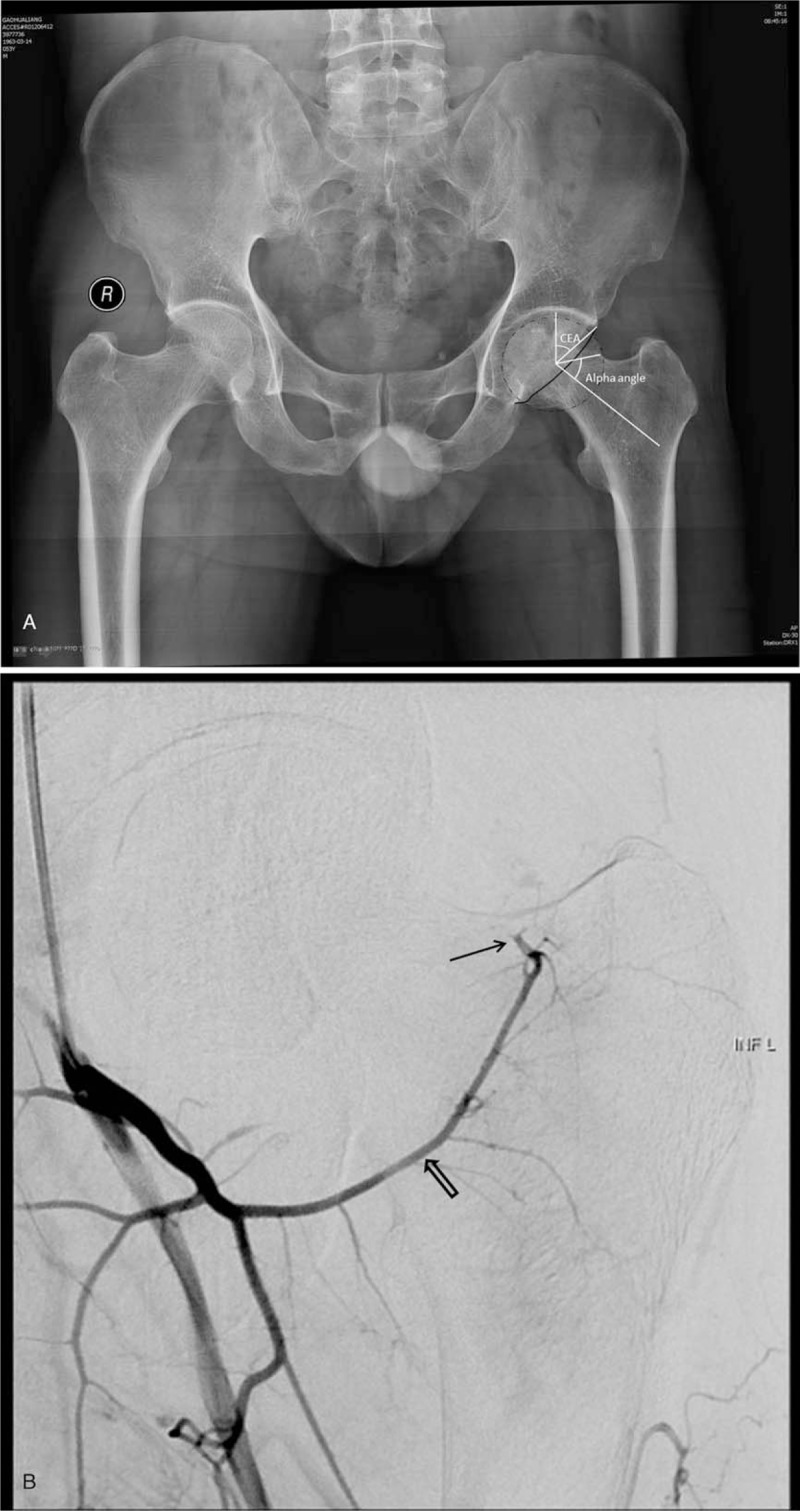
(A) X-ray image of a 20-year-old male patient. (B) DSA examination of the same patient in (A).
